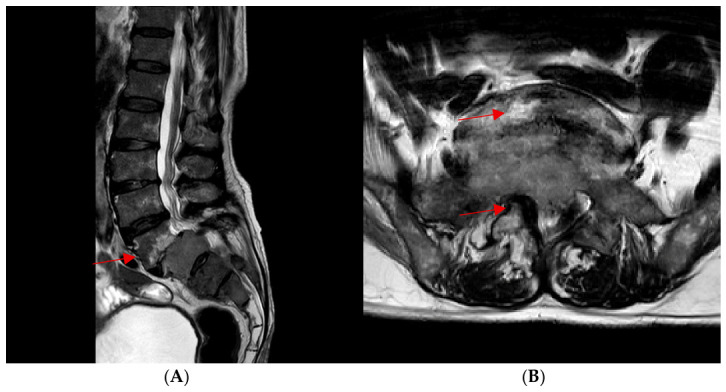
Lumbosacral MRI. (A) Sagittal T2-weighted image demonstrating an expansile lesion involving the S1–S3 segments with severe spinal canal stenosis and soft tissue extension. (B) Axial T2-weighted image confirming canal compromise and mass effect on the cauda equina. Arrows highlight the lesion and neural compression.
